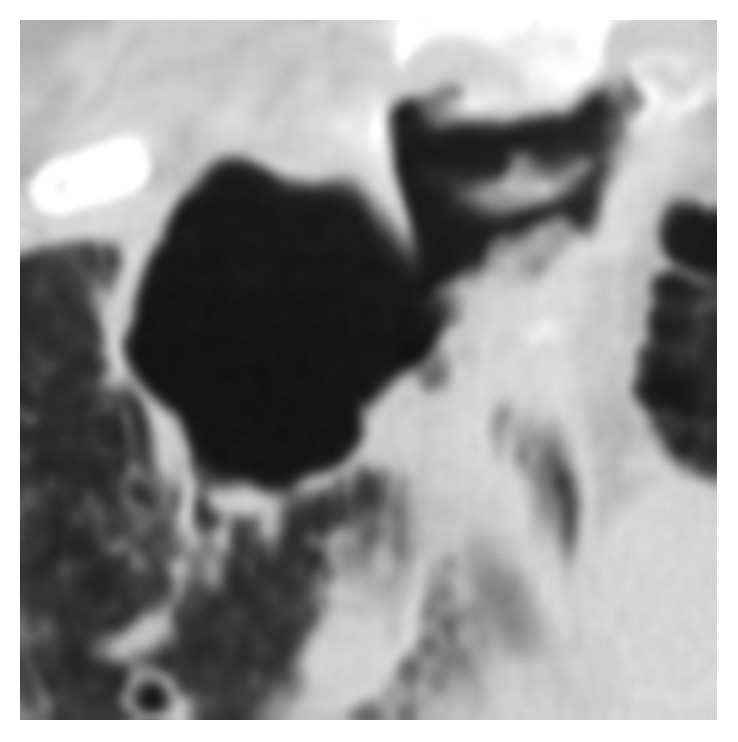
CT chest demonstrating contiguity of mass with bronchus.
